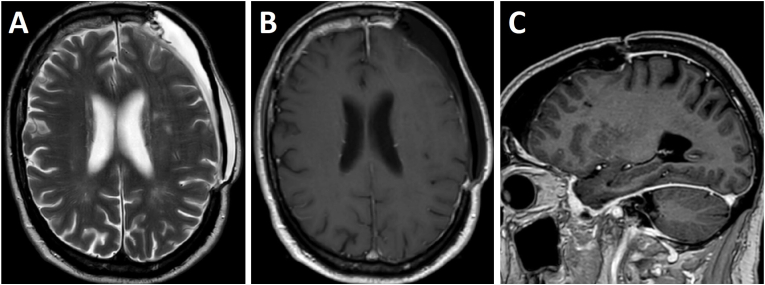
Postoperative Magnetic Resonance Imaging (MRI) at three months. Axial T2 (A) and T1-contrast-enhanced (B) MRI showing complete removal of the tumor with diminished left-sided dural thickening and enhancement extending to the basal dura mater (sagittal view in C).
